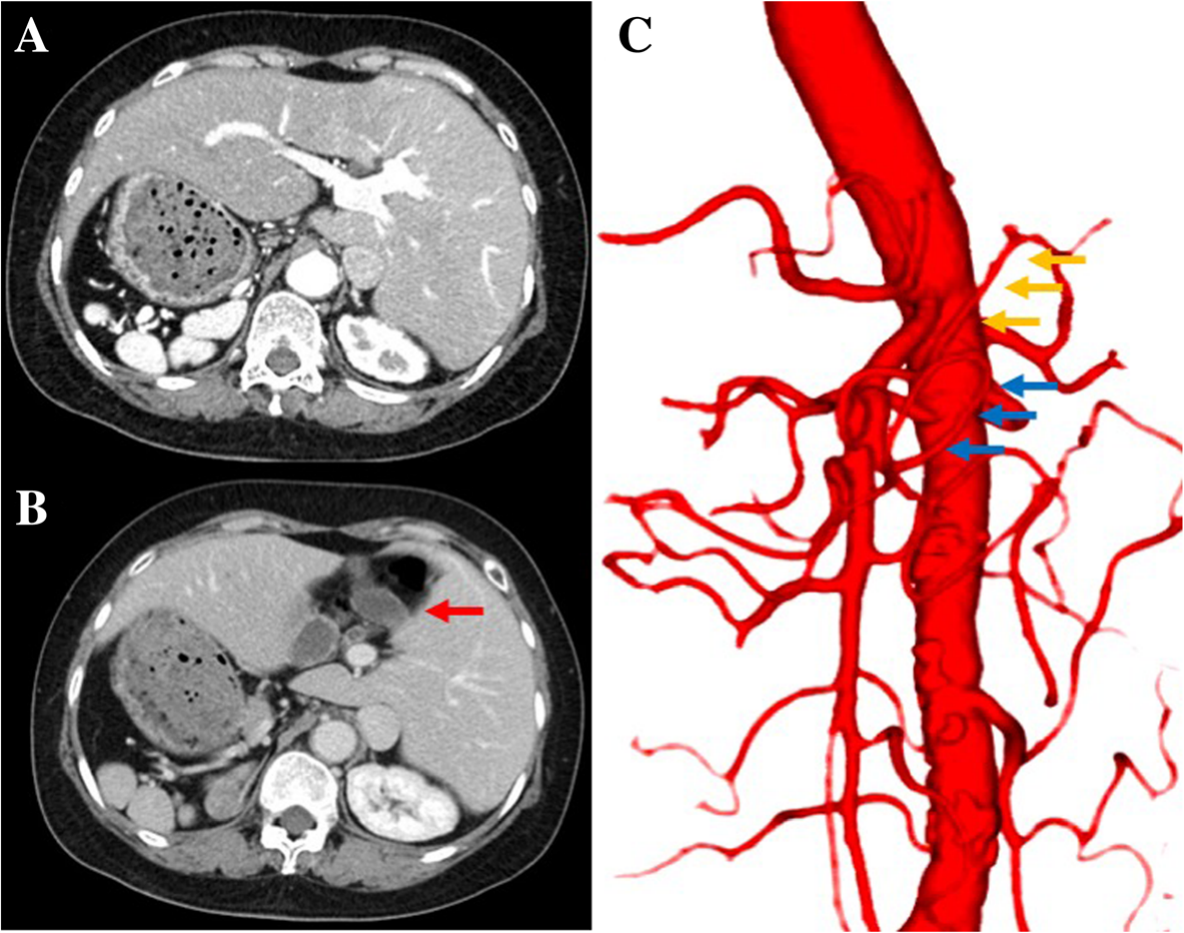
Computed tomographic (CT) image, preoperative three-dimensional CT angiography. a, b CT images showing polysplenia and left-sided gallbladder (*red arrow*). c Three-dimensional CT angiography showing the common hepatic artery (*yellow arrows*) and right gastroepiploic artery (*blue arrows*) arising from the first jejunal artery
